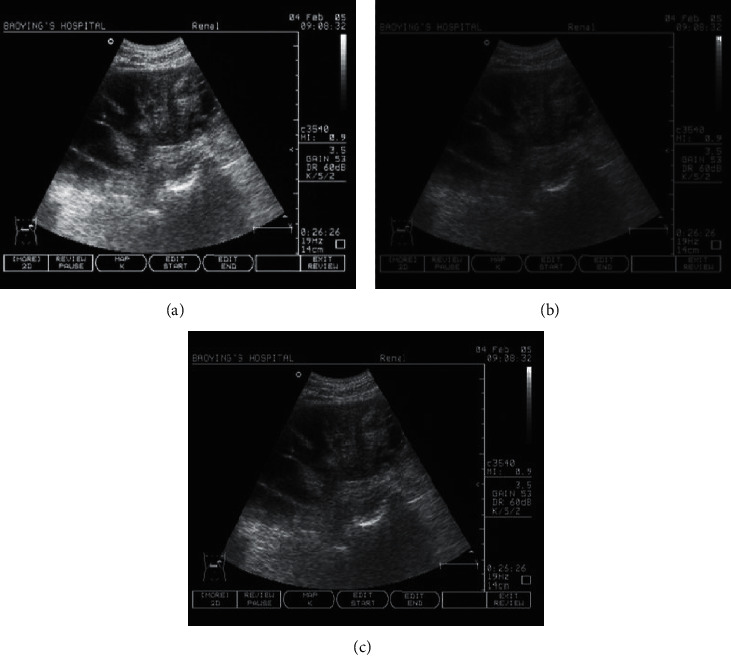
The ultrasound images of (a) histogram equalization, (b) linear transformation algorithm, and (c) artificial intelligence PCNN algorithm.
